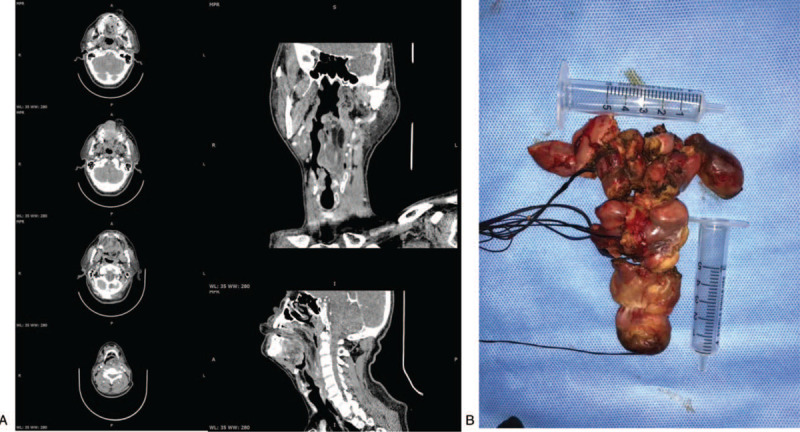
A. A high-definition CT scan showed that the tumor extending from left pyriform to oral cavity, the size of tumor was 2.38 × 2.78 × 16.86 cm. B. Because the tumor size was too large, the surgeon resected the tumor in stages with a Groff knife. The tumor was solid and rich in structurally abnormal vascular structure. CT = computed tomography.
